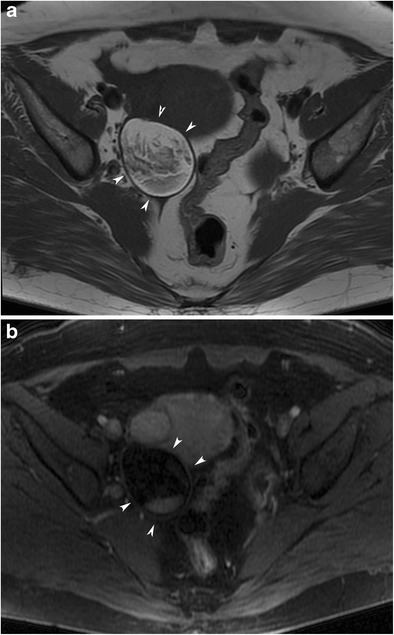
Axial T1-weighted MR image of a 42-year-old female with mature cystic teratoma (a) shows a palm tree-like structure projecting into the high signal intensity mass (arrowheads). Fat-saturated contrast-enhanced T1-weighted image (b) demonstrates a suppressed fat component in the tumour (arrowheads)
